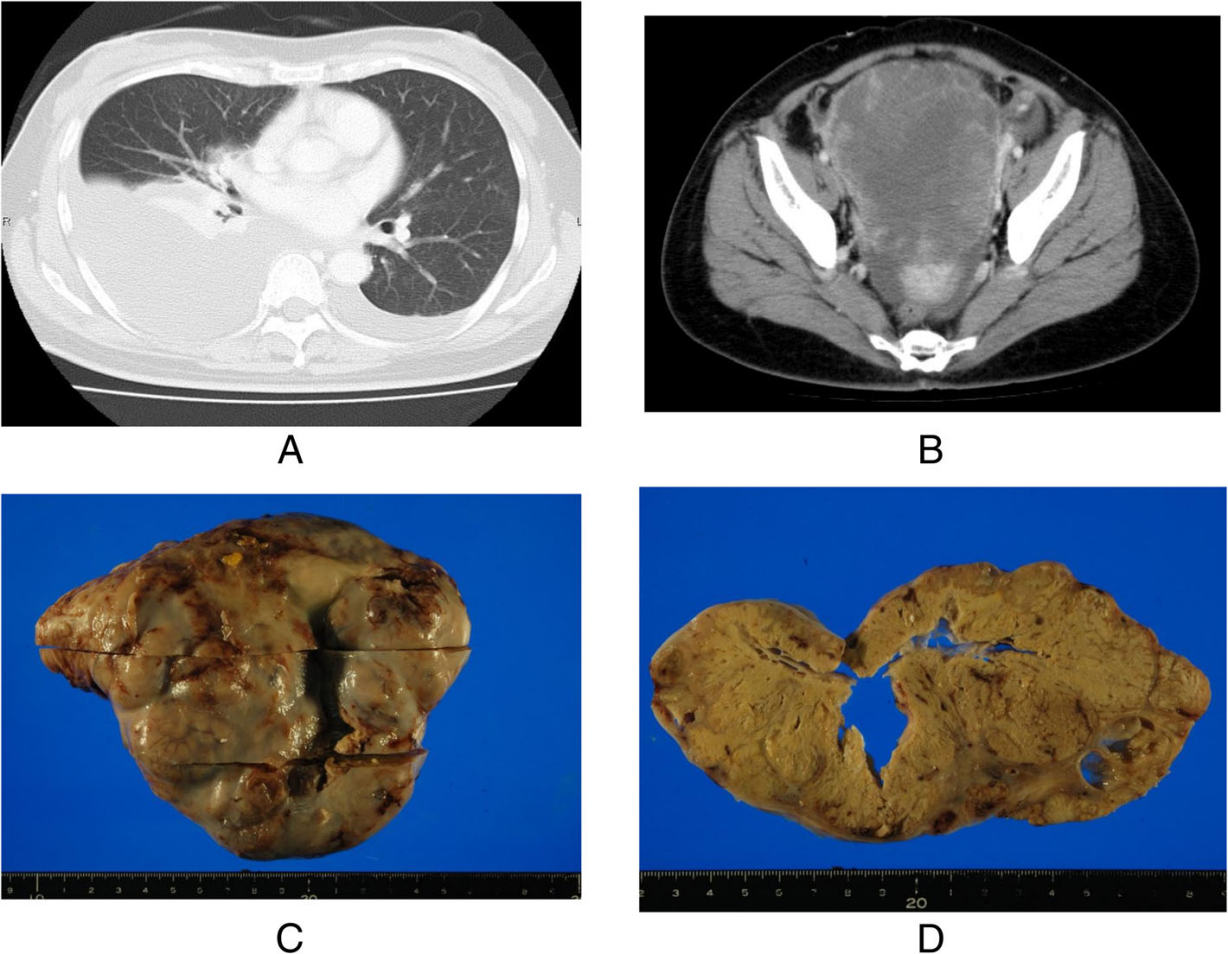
a Chest CT revealed massive pleural effusion at the right side and a small amount of left-side pleural effusion. b Abdominal CT indicated a large pelvic tumor. c The tumor of the left-side ovary measured 17 × 14 cm and contained necrotic tissues (d)
